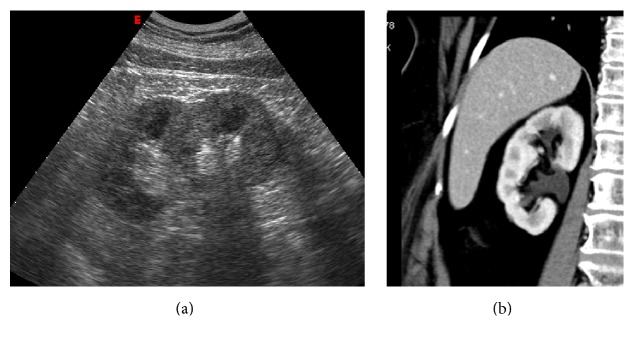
Persistent fetal lobulations. US image (a) and multiplanar coronal contrast-enhanced CT obtained in corticomedullary phase (b). The typical appearance of the normal congenital variant is depicted on both US and contrast-enhanced CT.
